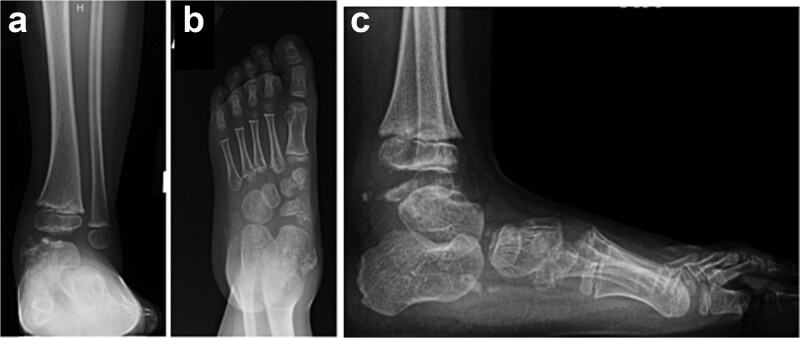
AP ankle (a), AP foot (b), and lateral ankle (c) radiographs demonstrating osteochondral irregularity of the talar dome with disruption of the ankle mortise and flattening of the medial longitudinal arch.
